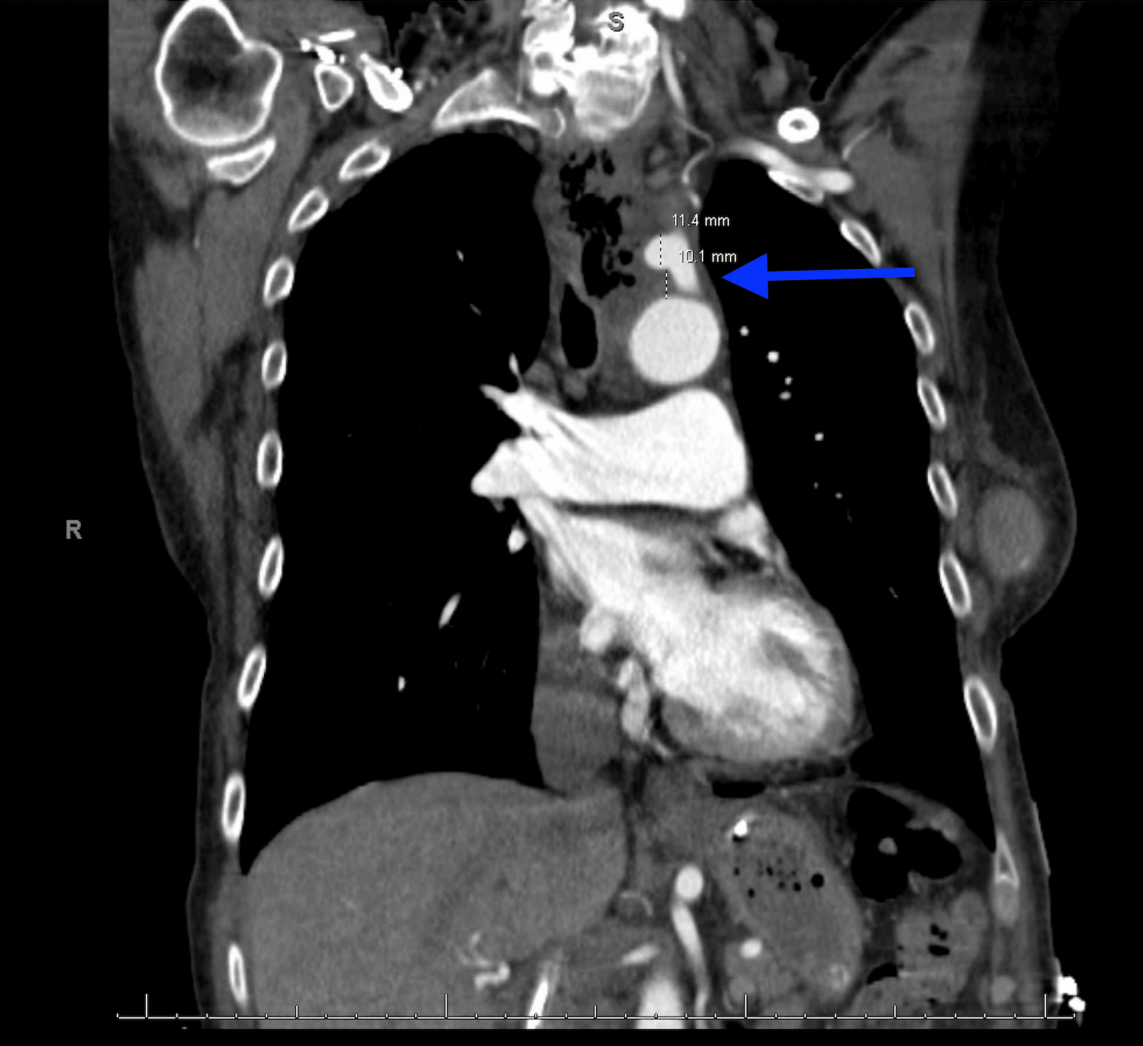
Left subclavian artery pseudoaneurysm due to tumor invasion. Coronal CT image showing a left subclavian artery pseudoaneurysm (blue arrow) measuring 1.2 cm × 1.1 cm × 1.3 cm, located approximately 1.2 cm from its origin off the aortic arch.
